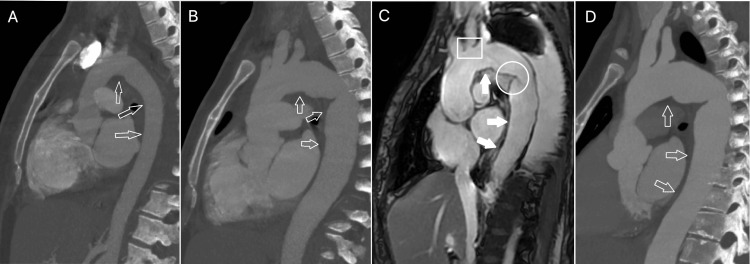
Evolution of aortic involvement in Takayasu arteritis. (A) Sagittal thoracic CT angiography (bone window) showing standard aortic caliber (arrows). (B) Repeat CT angiography 22 days later, demonstrating diffuse thickening of the aortic arch, including both ascending and descending segments (arrows). (C) Chest magnetic resonance angiography (MRA) confirming concentric wall thickening of the thoracic aorta. A square highlights stenosis of the ostium of the right common carotid artery, and a circle denotes an intimal flap in the distal thoracic aorta. (D) Follow-up CT angiography performed six weeks later shows moderate improvement in aortic thickening.
